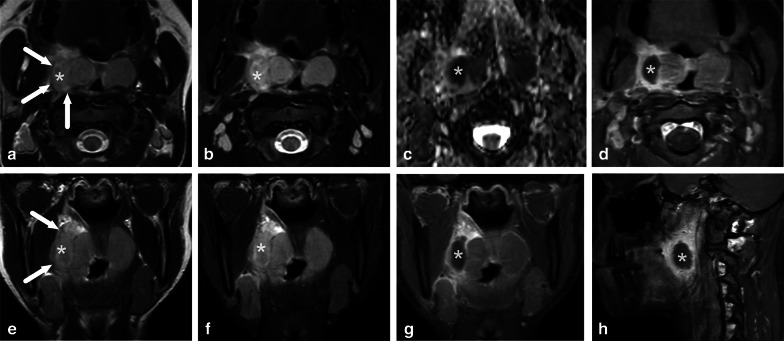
Lateral uncomplicated PTA in a patient with a sore throat. Axial and coronal T2-weighted images without (a, e) and with (b, f) fat suppression demonstrate an abnormal collection (asterisk) with intermediate T2-signal between the tonsil and a swollen pharyngeal constrictor muscle (arrows). Axial (d), coronal (g), and sagittal (h) fat-suppressed post-contrast T1-weighted images show a centrally non-enhancing fluid collection, and low ADC (c) suggests purulence. PTA was found upon tonsillectomy
